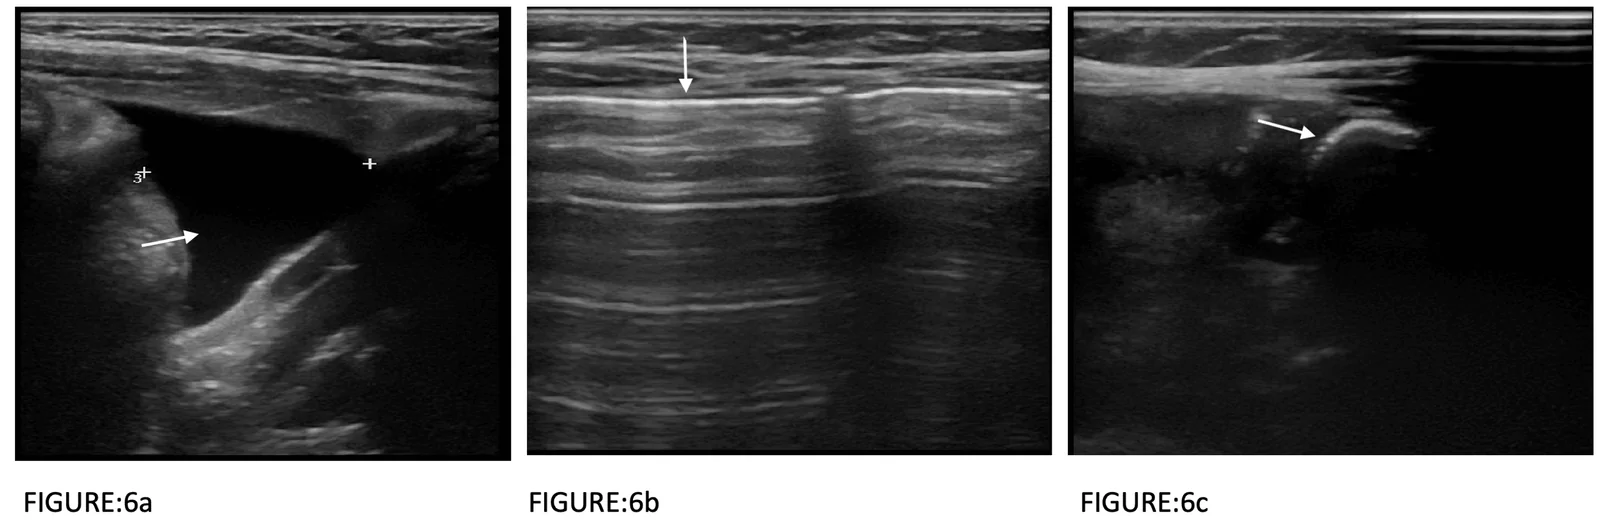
(a) A small amount of free fluid in the right lower abdomen (white arrow), (b) Pneumoperitoneum (white arrow), (c) Pneumatosis (white arrow), better evaluated on CT scan abdomen
